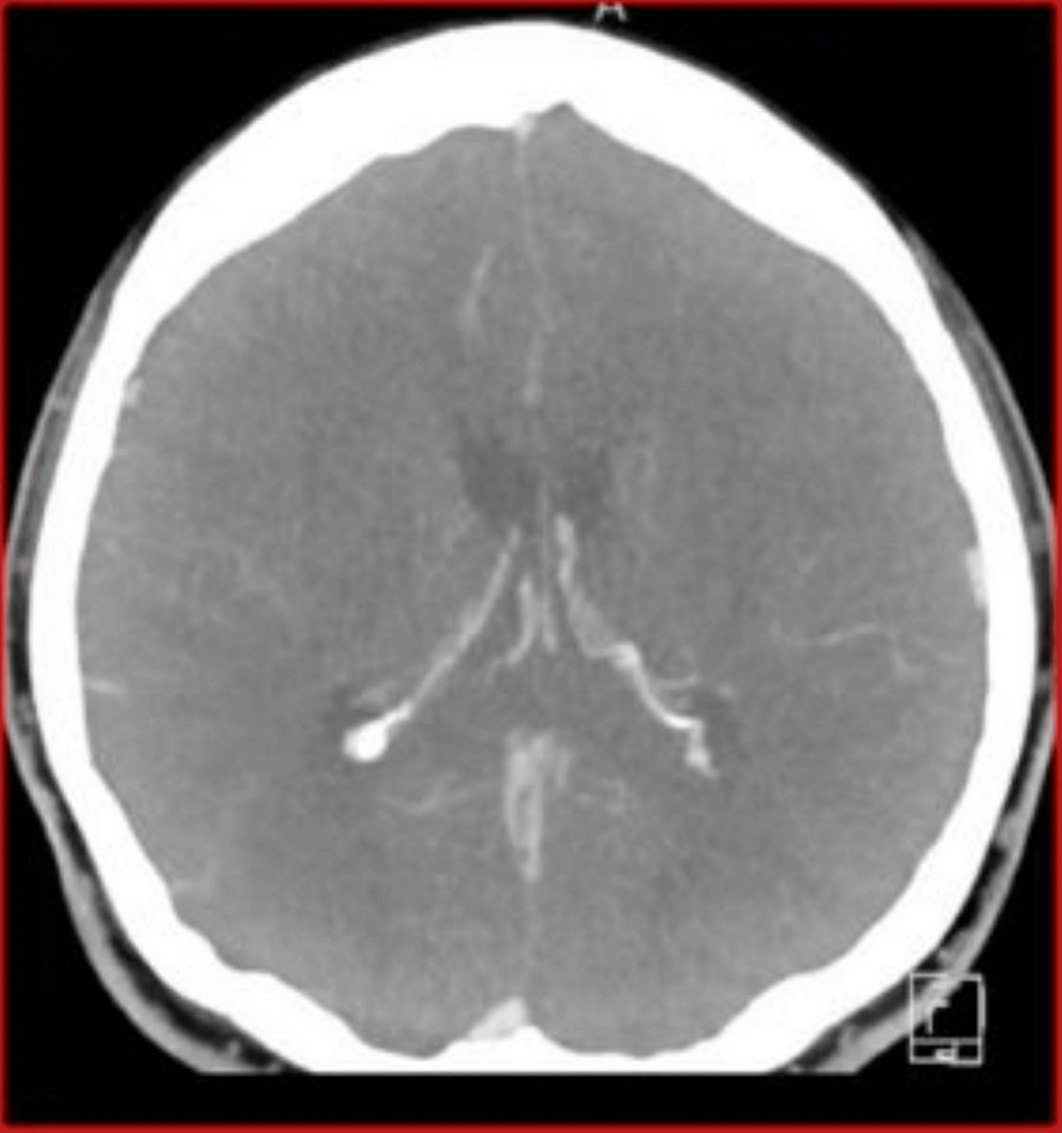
CT venogram with no blockages identified
